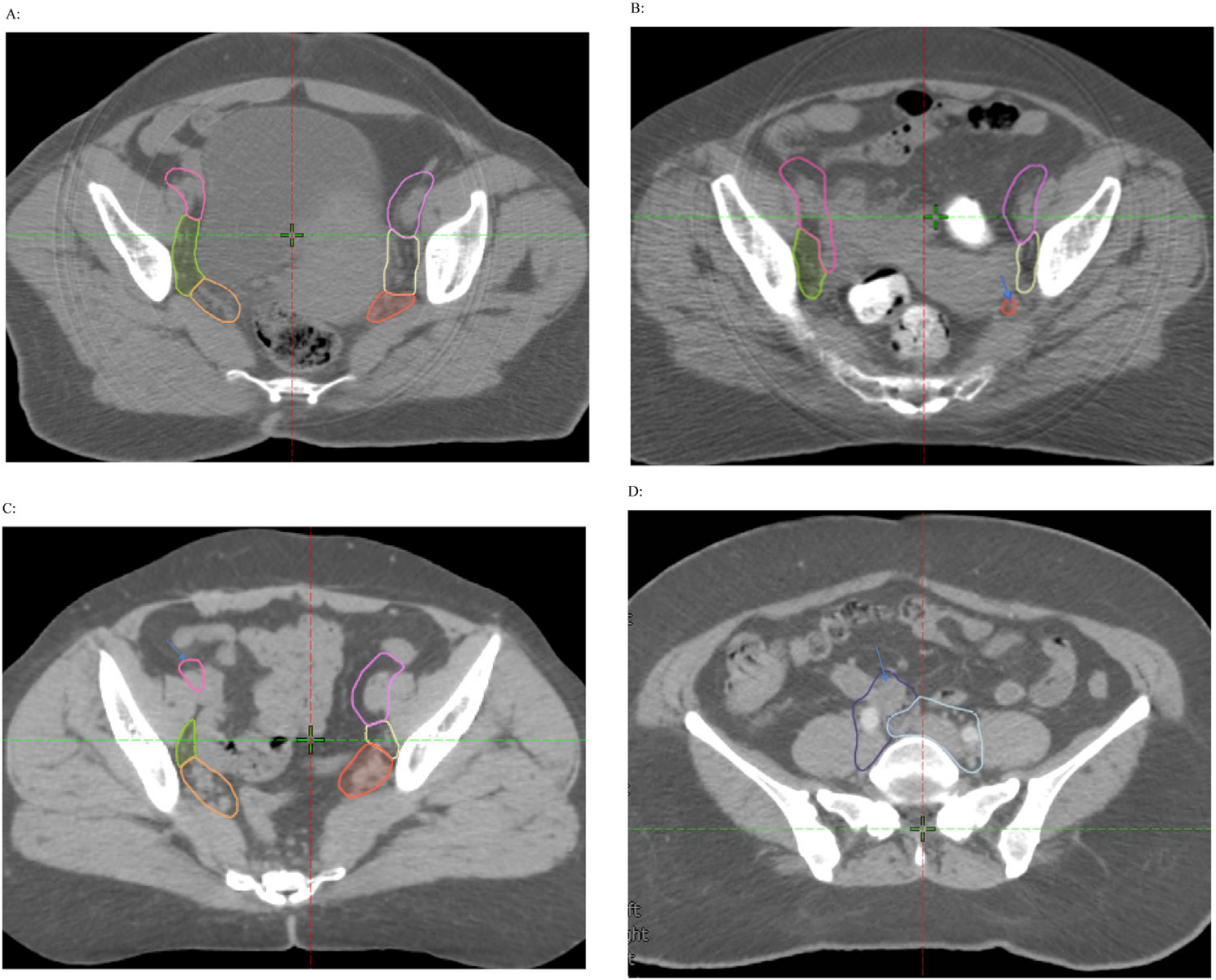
Representative axial computed tomography slices for female patients showing auto-segmented pelvic nodal regions. (A) Scored 4, requiring no edits at all. (B) Scored 2, missing internal iliac nodal region (arrow). (C) Scored 3, cutting through external iliac nodal region (arrow) in three or fewer slices. (D) Scored 3, bowel loop (arrow) included in common iliac in 2 slices.
